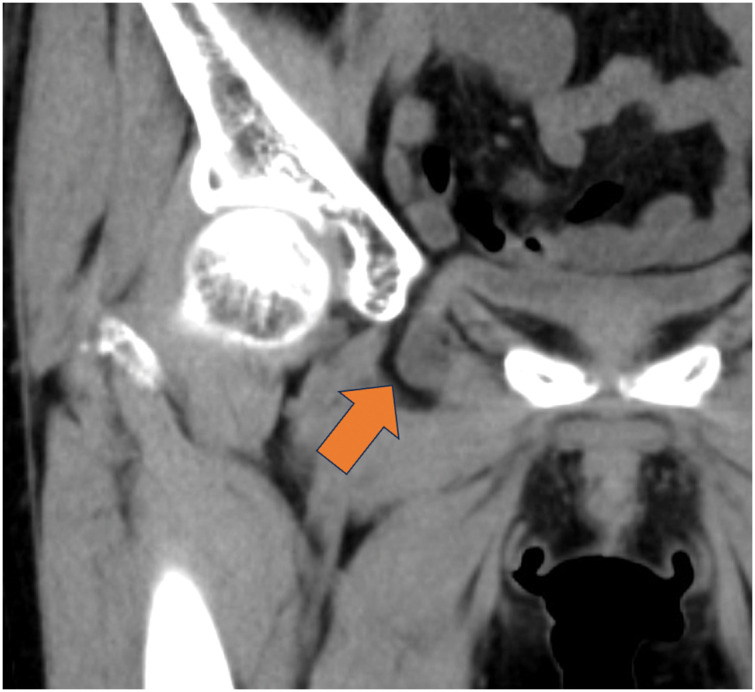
The CT scan performed at our hospital. Arrow: bladder herniation through the obturator foramen.
